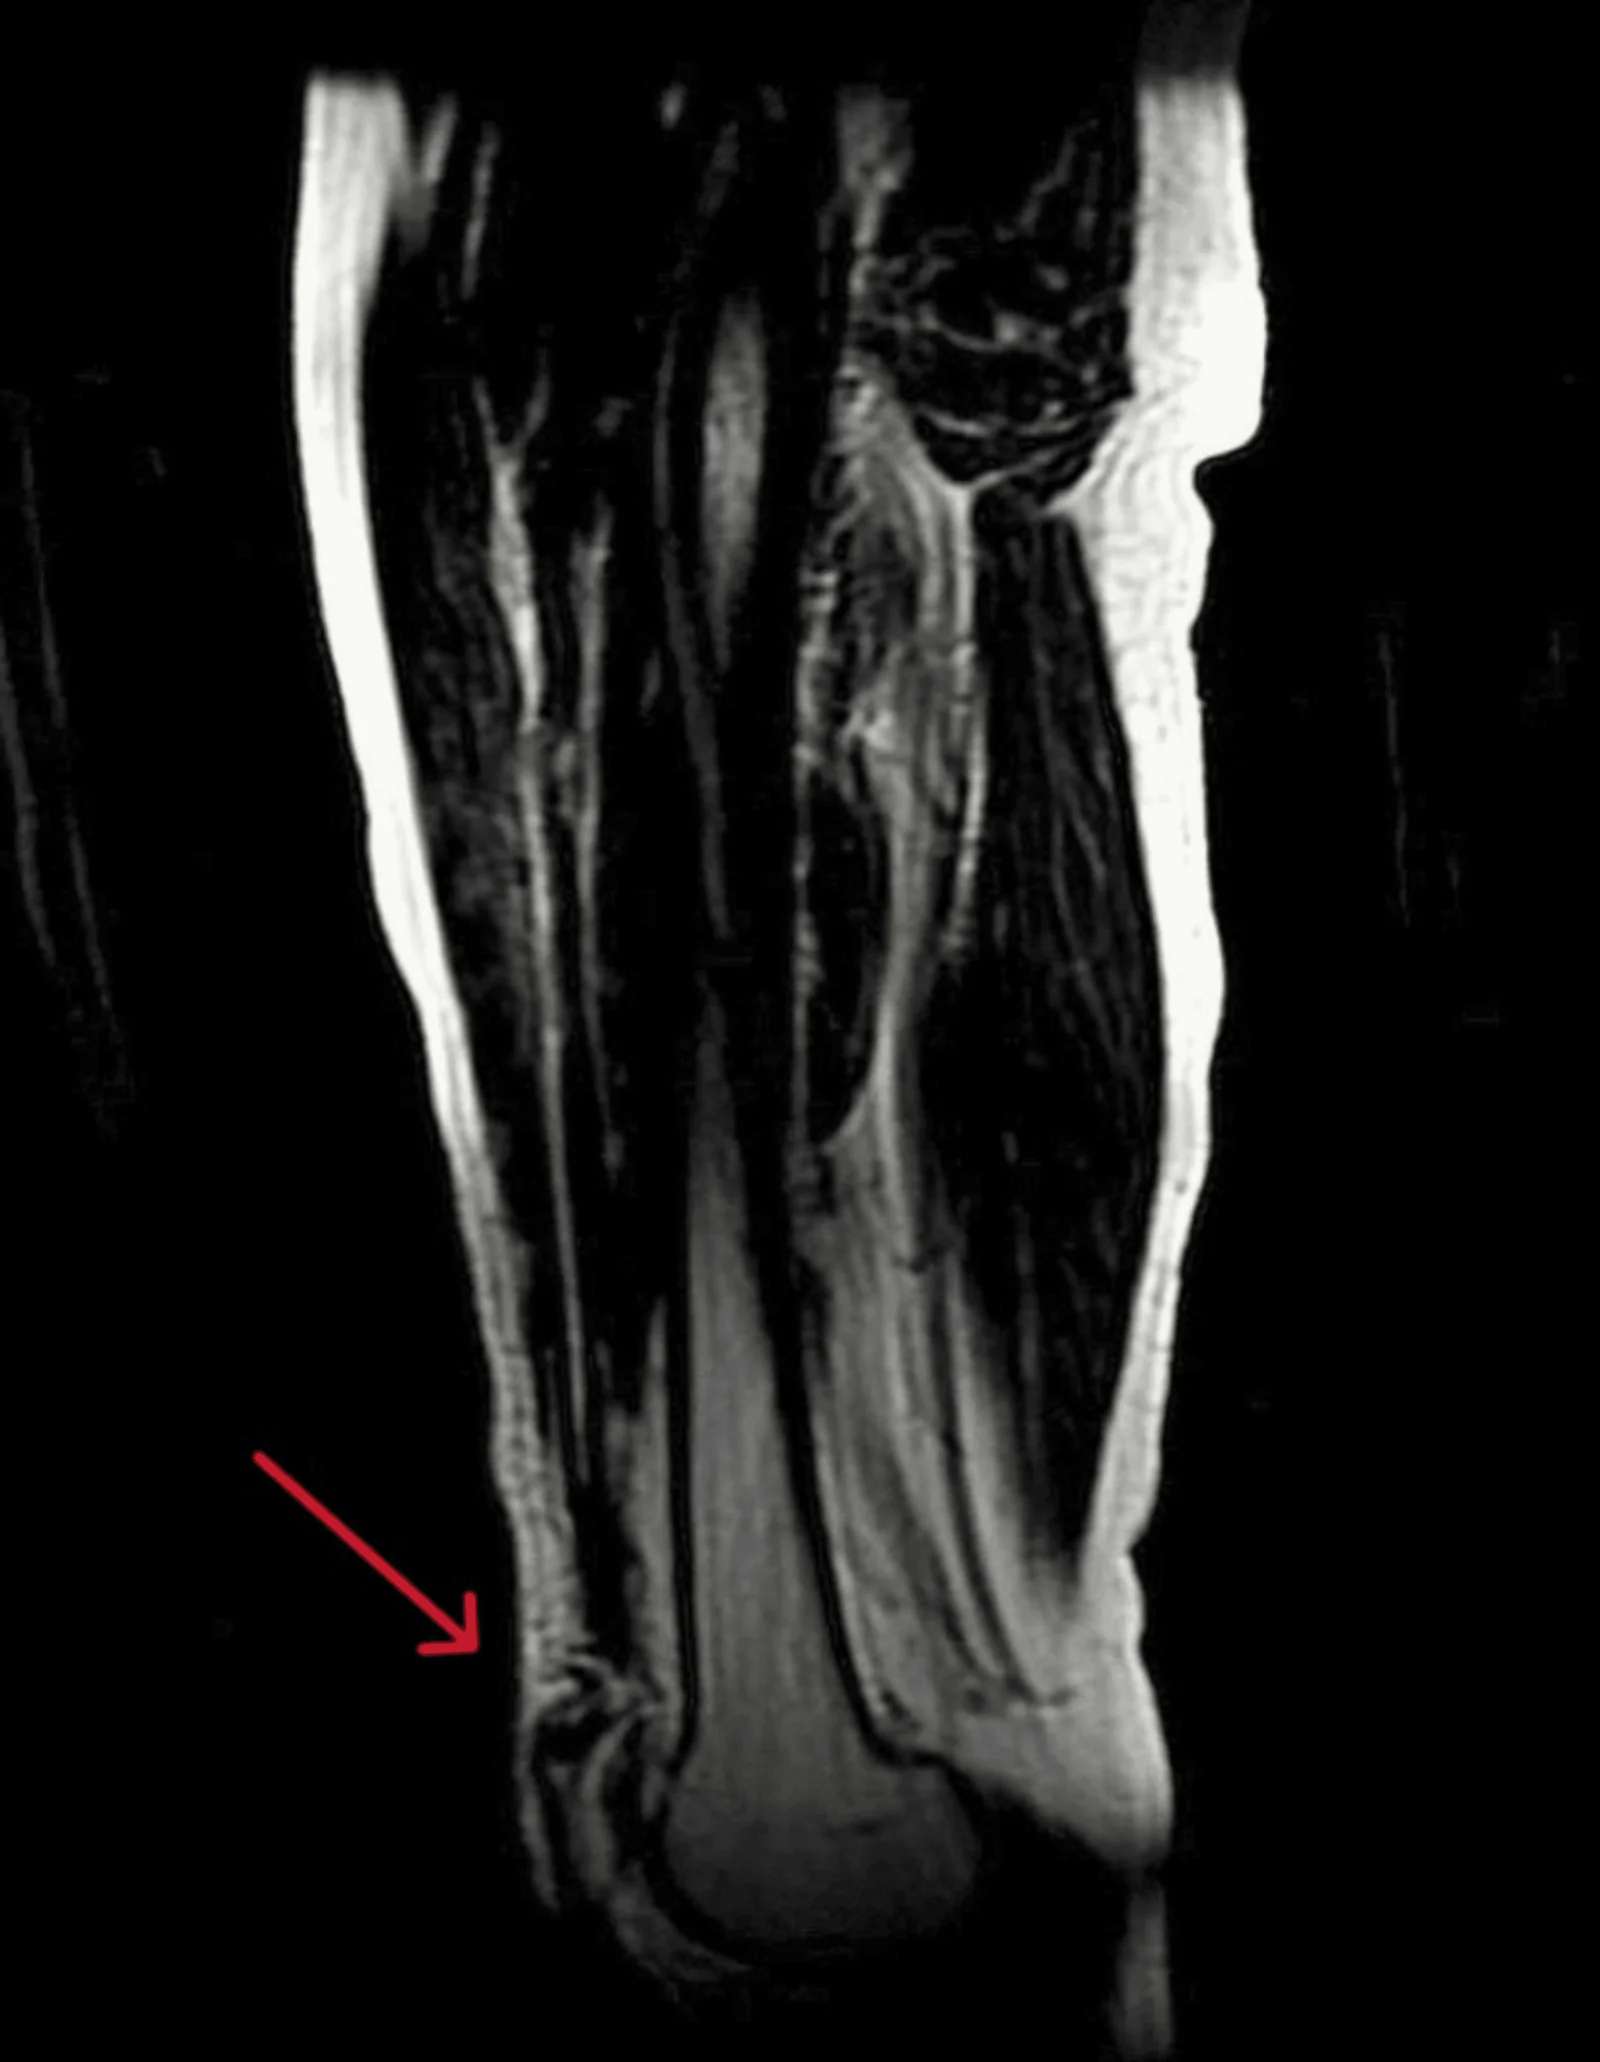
Sagittal T2-weighted MRI of the right knee demonstrating discontinuity of the quadriceps tendon at its insertion on the superior pole of the patella (arrow).
